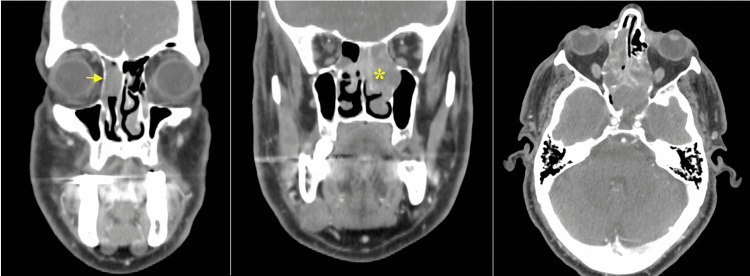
A contrasted CT scan of the paranasal sinuses showing a faintly enhancing soft-tissue mass in the right nasal cavity (arrow) with extension to the left nasal cavity, right frontal sinus, bilateral ethmoidal sinuses, left maxillary wall, and left sphenoid (asterisk).
